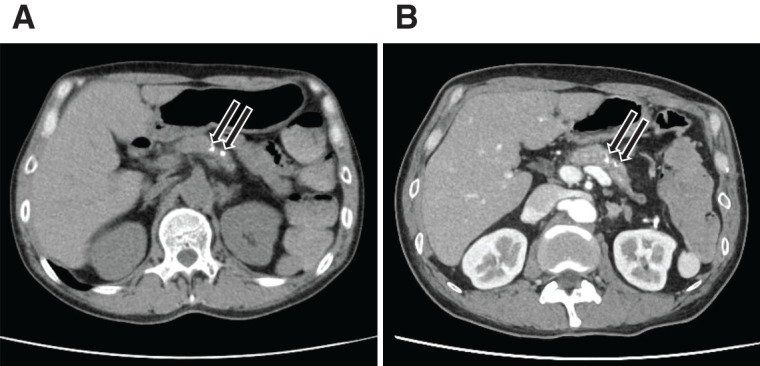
Preoperative CT imaging. (A) Unenhanced CT reveals hyperdense areas within the pancreas suggestive of calcifications (arrows). (B) Contrast-enhanced CT shows the same hyperdense areas at the corresponding site (arrows), which is also suggestive of pancreatic calculi, with no evidence of active extravasation.
